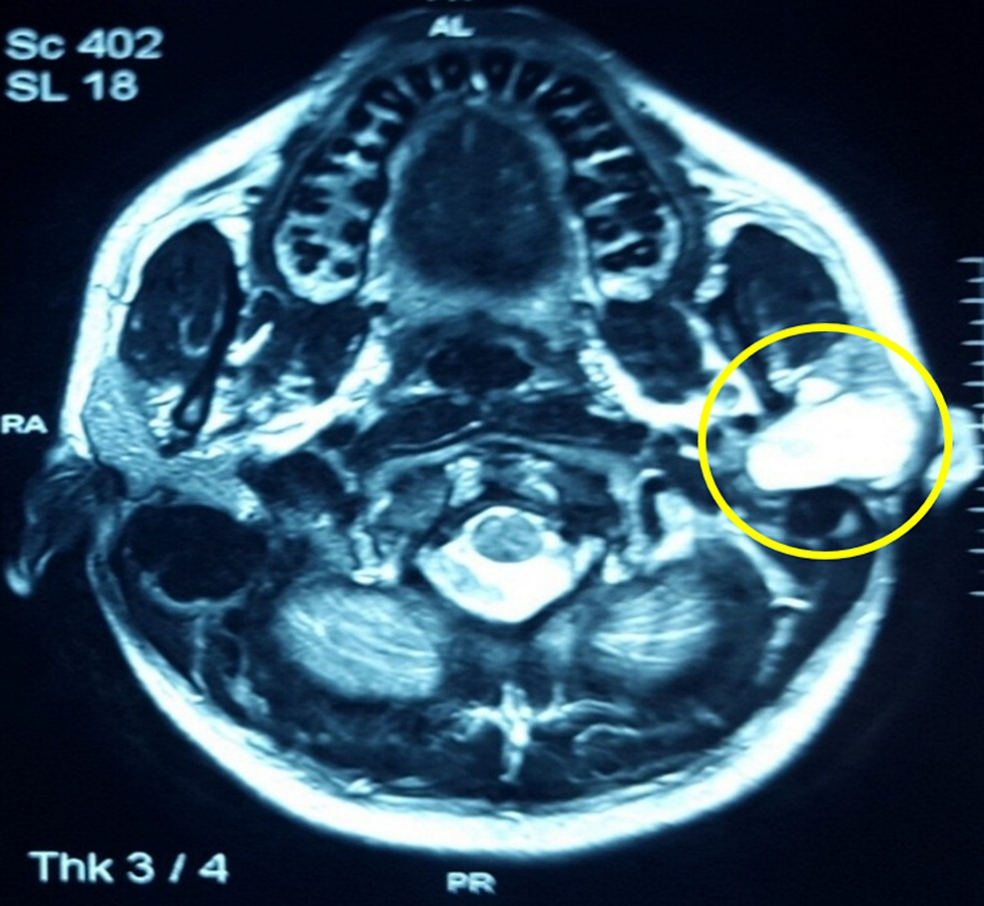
Preoperative axial sections of the MRI scan.
